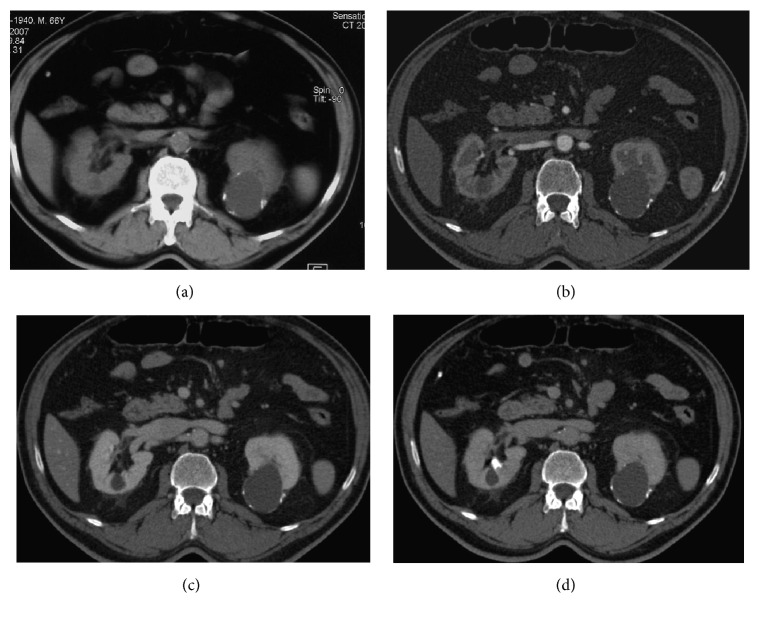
Bosniak category IIF cyst. Axial unenhanced (a) and enhanced CT-scans performed at corticomedullary (b), nephrographic (c), and excretory phase (d). The images show a large hypodense cyst in the left kidney with wall calcifications. A small simple cyst is also visible in the third middle of the right kidney.
